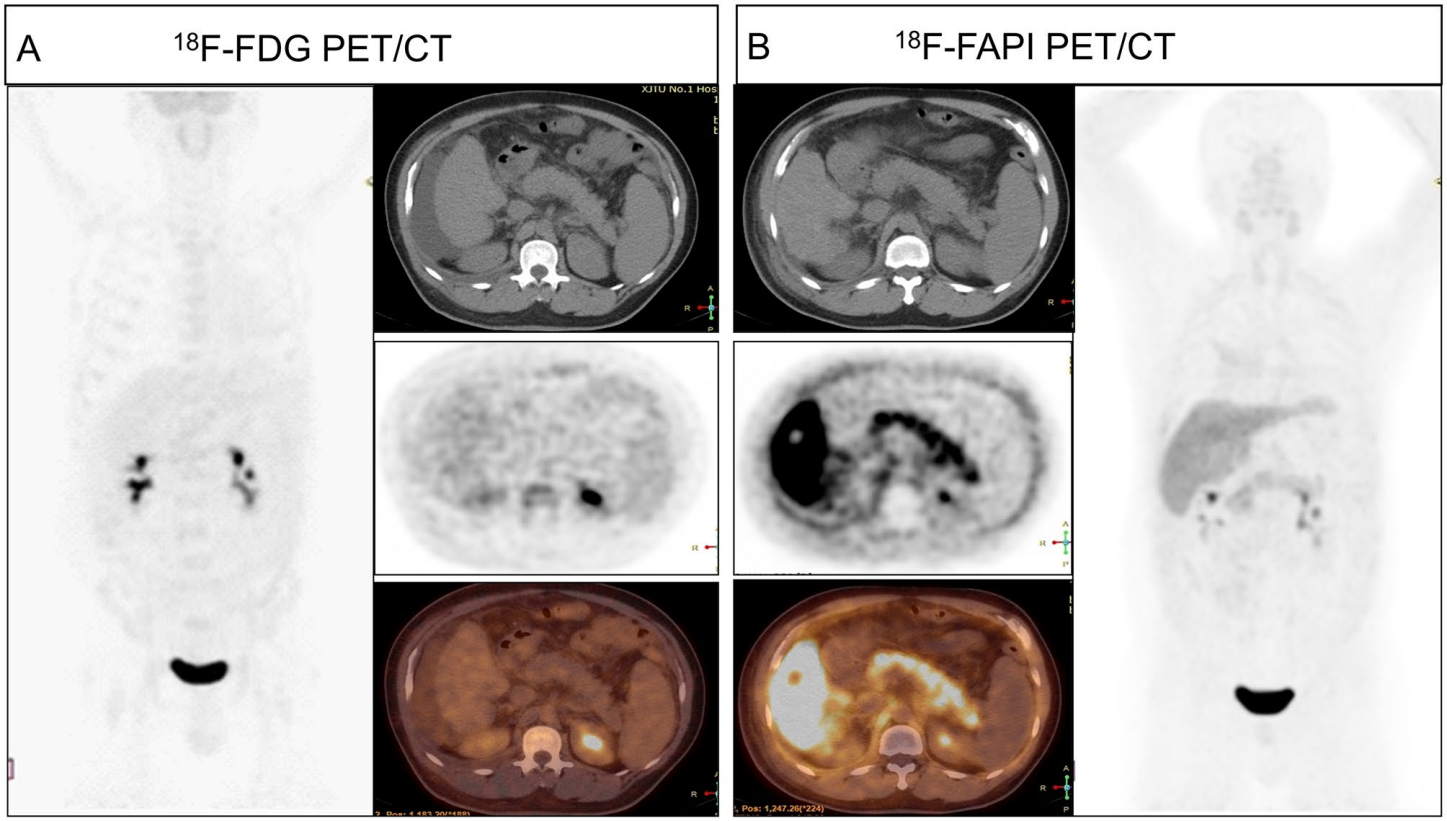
NSU in the ^18^F-FAPI of the cirrhosis of the liver and negative in the ^18^F-FDG PET/CT. A male patient, 42 years of age, with chronic cirrhosis of the liver. ^18^F-FDG PET/CT (A) showed no FDG concentration in the pancreas, and ^18^F-FAPI PET/CT imaging (B) showed a high concentration in the pancreas, with an SUVmax of 6.3. *FAPI* fibroblast activation protein, *FDG* fluorodeoxyglucose, *PET/CT* positron emission tomography/computed tomography, *SUVmax* maximum standardized uptake value.
